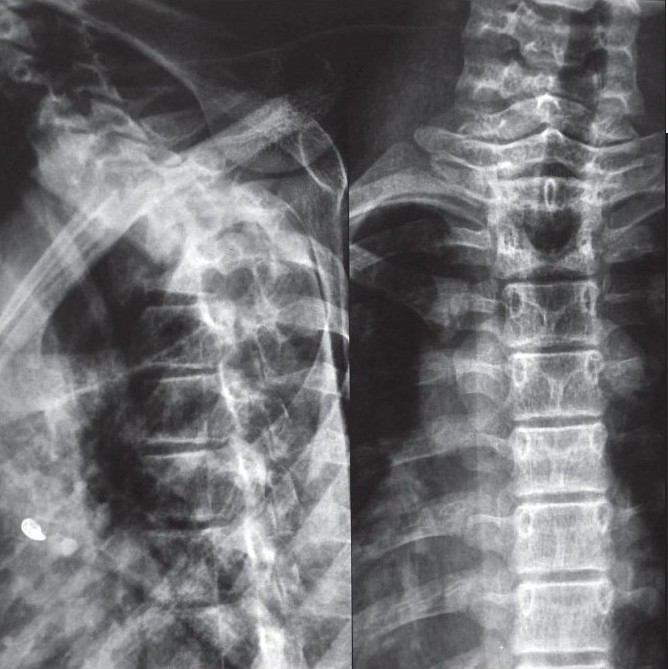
Lateral and anteroposterior X-rays of upper dorsal spine lesion show kyphosis of 80° of upper dorsal spine. Anteroposterior view shows end on appearance of D 1, 2, 3 with crowding of ribs
